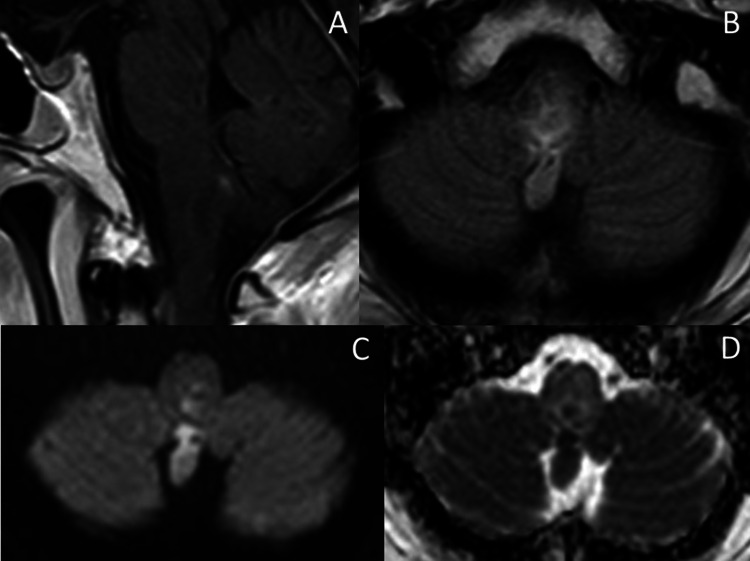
Rhombencephalitis with abscess. (A,B) Sagittal T1W and axial FLAIR with gadolinium enhancement at the posterior fossa demonstrate faint rim enhancement with T2/FLAIR hyperintense signal change at the medulla, including the obex, representing rhombencephalitis. (C,D) Axial DWI and ADC at the posterior fossa, and the content in the aforementioned rim-enhancing lesion at the medulla show restricted diffusion, which protrudes into the median aperture of the fourth ventricle, likely representing pus content.
